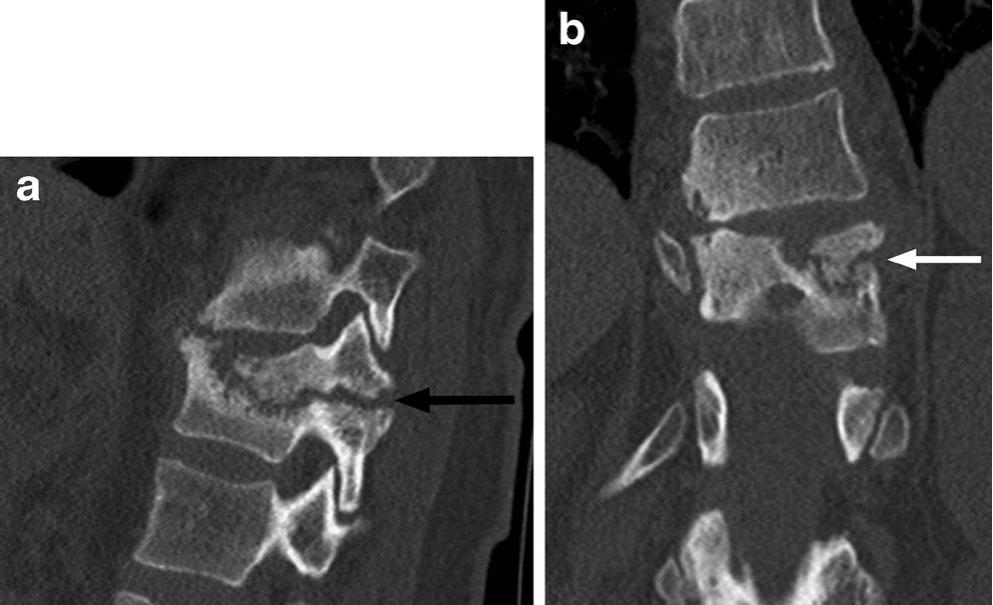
Charcot spine in a 32-year-old female with SCI due to a motor vehicle crash 2 years prior. a Parasagittal CT image of the left T11 pedicle shows development of an irregular pseudarthrosis with sclerosis and fragmentation (*black arrow*). b Coronal CT image depicts the pseudarthrosis (*white arrow*) and disc space narrowing above it. There is associated mild dextroscoliosis
